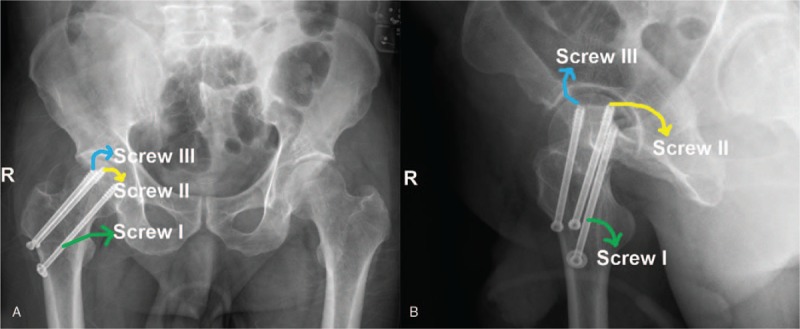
Postoperative x-ray of hip was examined. (A) Anteroposterior hip radiograph and (B) lateral hip radiograph.
